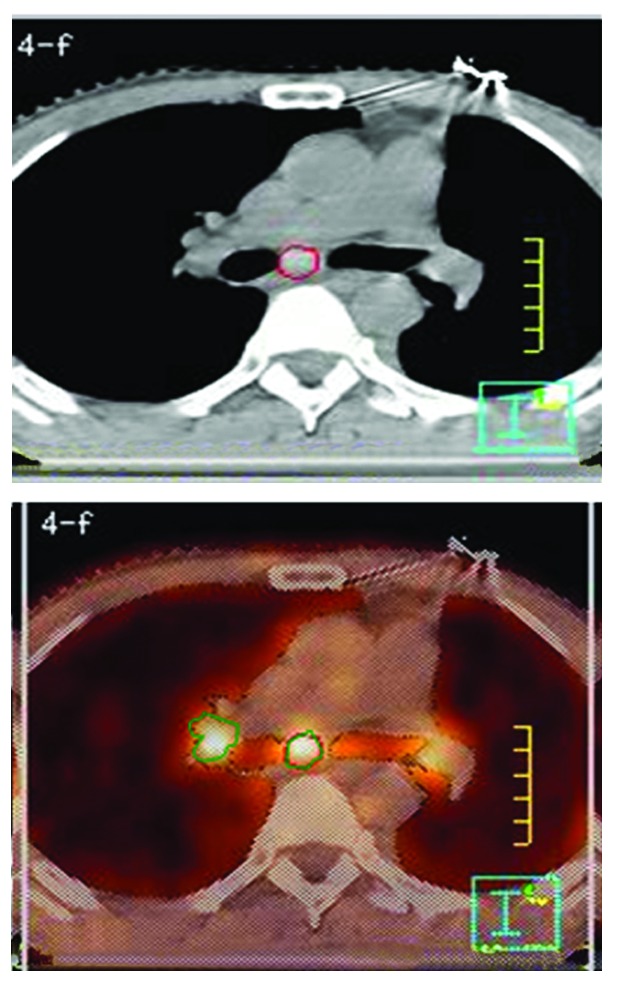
Alteration of gross tumor volume (GTV) by positron emission tomography/computed tomography (PET/CT) scan in one patient with unsuspected nodal disease. The red and green outlined areas indicate GTV based on the CT image and the PET/CT image, respectively.
